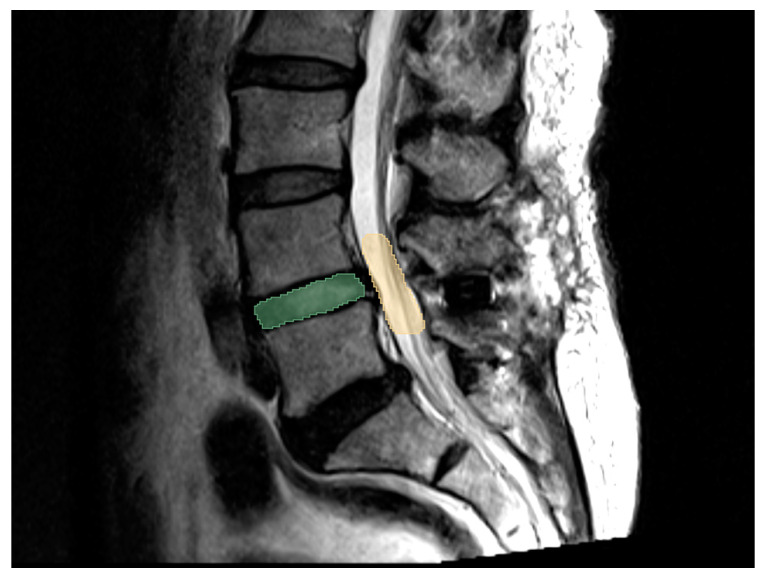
Three-dimensional volume measurement of Magnetic Resonance Imaging (MRI) via two-dimensional reconstruction sagittal image. Disc volume was measured between vertebral endplates as green area. Foraminal volume was measured between midportion of pedicles at adjacent levels as yellow area.
